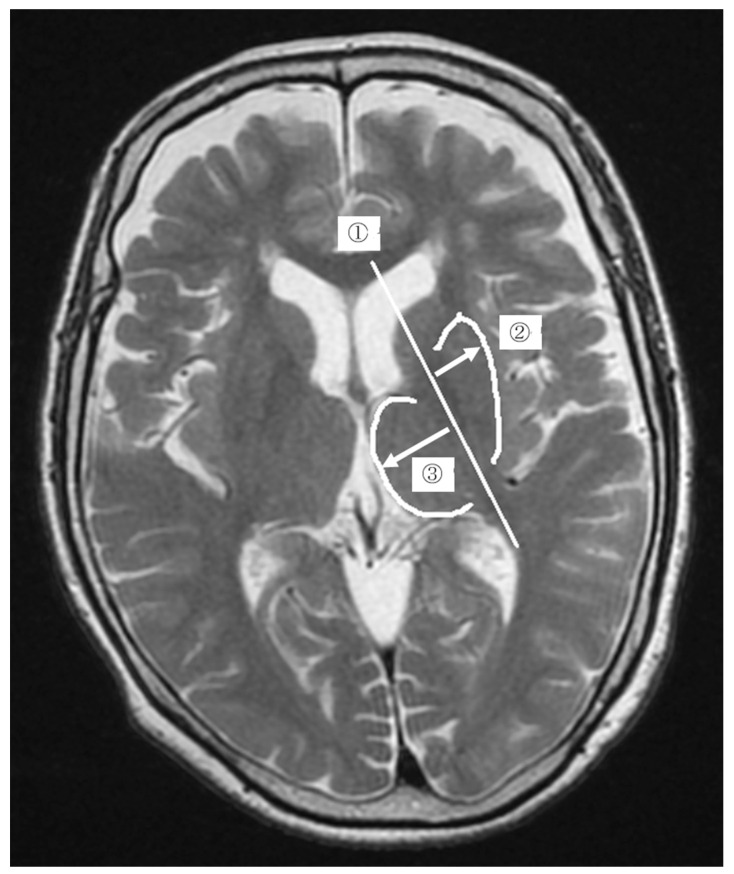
The measurement of the change ratio in the putamen using magnetic resonance images. First, we drew a straight line in the center of the posterior limb of the internal capsule and named it “the posterior limb of the internal capsule line” ➀. Second, we measured the distance from the posterior limb of the internal capsule line to the distal outline of the putamen (Right-pointing white arrow) and named it “putamen distance” ➁. Third, we measured the distance from the posterior limb of the internal capsule line to the distal outline of the thalamus (Left-pointing white arrow) and named it “thalamus distance” ➂. Finally, the ratio of putamen distance to thalamus distance was calculated, and the value was defined as the “change ratio in the putamen”.
